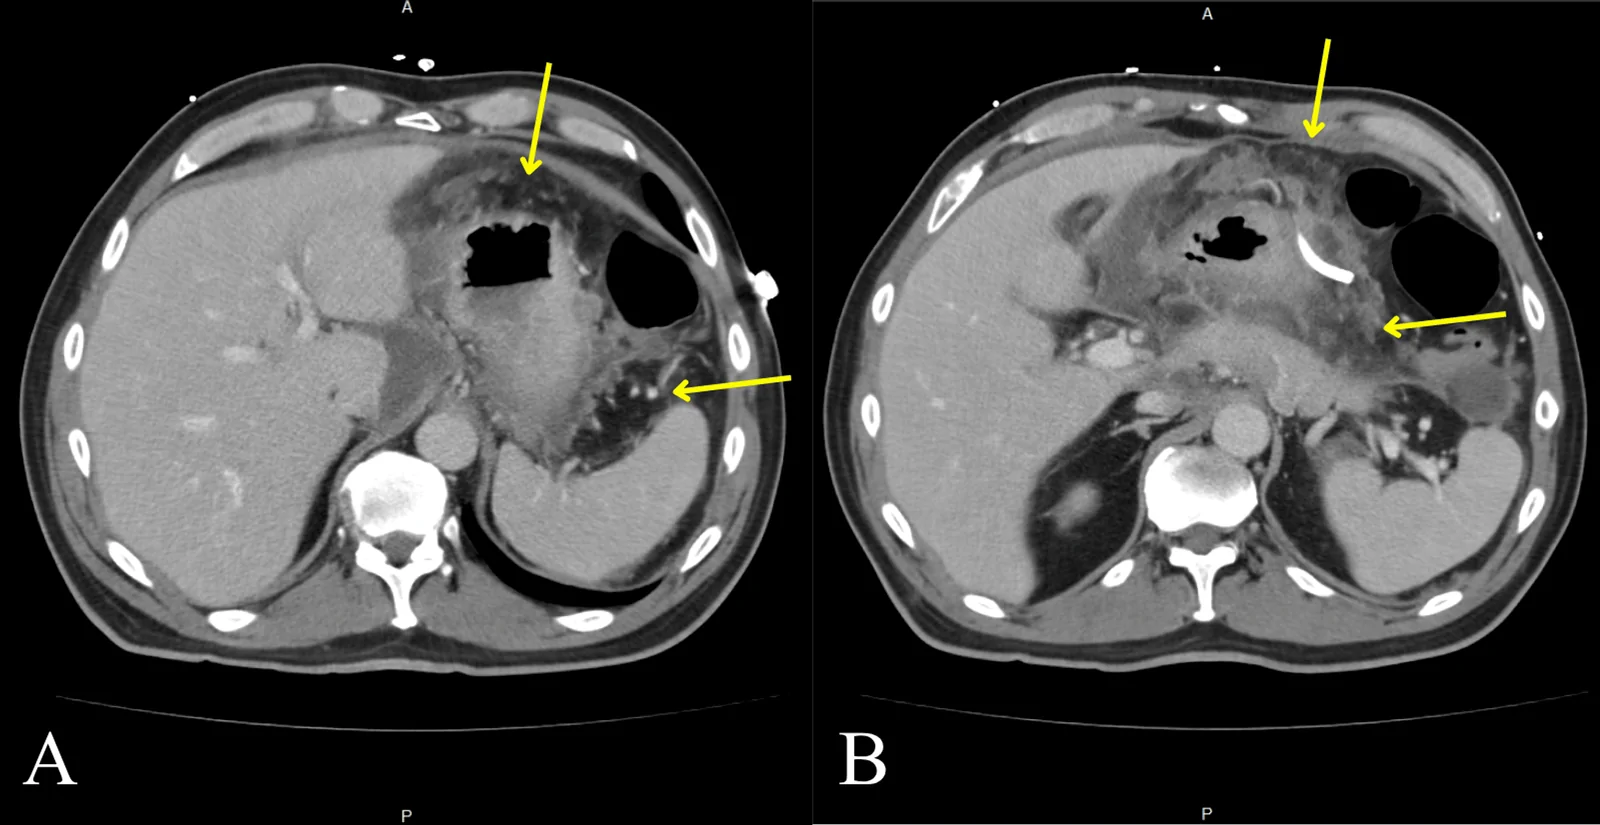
Axial contrast-enhanced CT of the upper abdomen demonstrating appearances consistent with sequelae of pancreatitis with sterile peripancreatic necrosis Axial contrast‑enhanced CT of the upper abdomen (panel A) demonstrating heterogeneous low‑attenuation peripancreatic collections (upper arrow) in the lesser sac posterior to the stomach, with extension of fluid and necrotic tissue along the medial aspect of the spleen (lower arrow), consistent with sterile peripancreatic necrosis and organized fluid collections in the setting of pancreatitis. Axial contrast‑enhanced CT of the upper abdomen (panel B) demonstrating heterogeneous low‑attenuation peripancreatic collections replacing the expected pancreatic contour. The superior arrow indicates necrotic fluid and soft tissue extending anteriorly from the pancreatic bed, while the inferior arrow highlights a more focal peripancreatic collection posterior to the stomach, consistent with organized sterile peripancreatic necrosis in pancreatitis.
